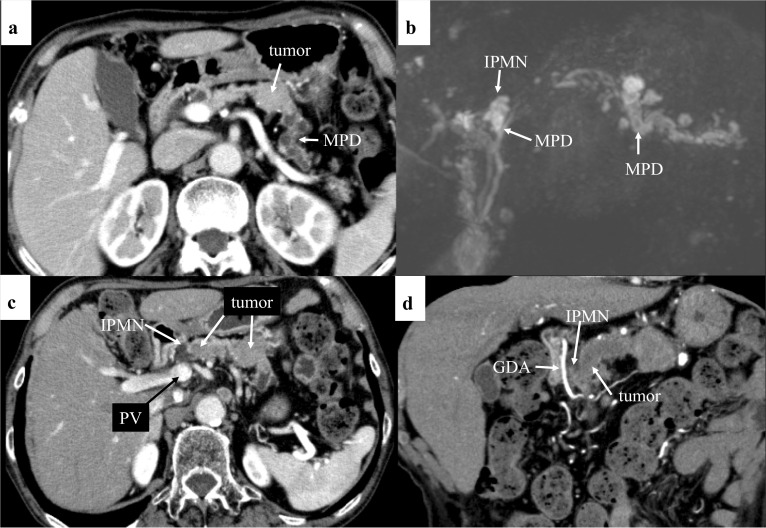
a Multi-detector row computed tomography (MDCT) shows a slightly enhanced round tumor and the dilated caudal main pancreatic duct (MPD). b Magnetic resonance cholangiopancreatography (MRCP) demonstrates mild dilatation of cranial MPD and severe dilatation of caudal MPD. Cystic lesion [suspicious intraductal papillary mucinous neoplasm (IPMN)] is visible in the neck of the pancreas. c MDCT shows a tumor with downstream progression until the left side of IPMN. d A coronal view shows intraductal tumor mass extends to the left side of the IPMN
